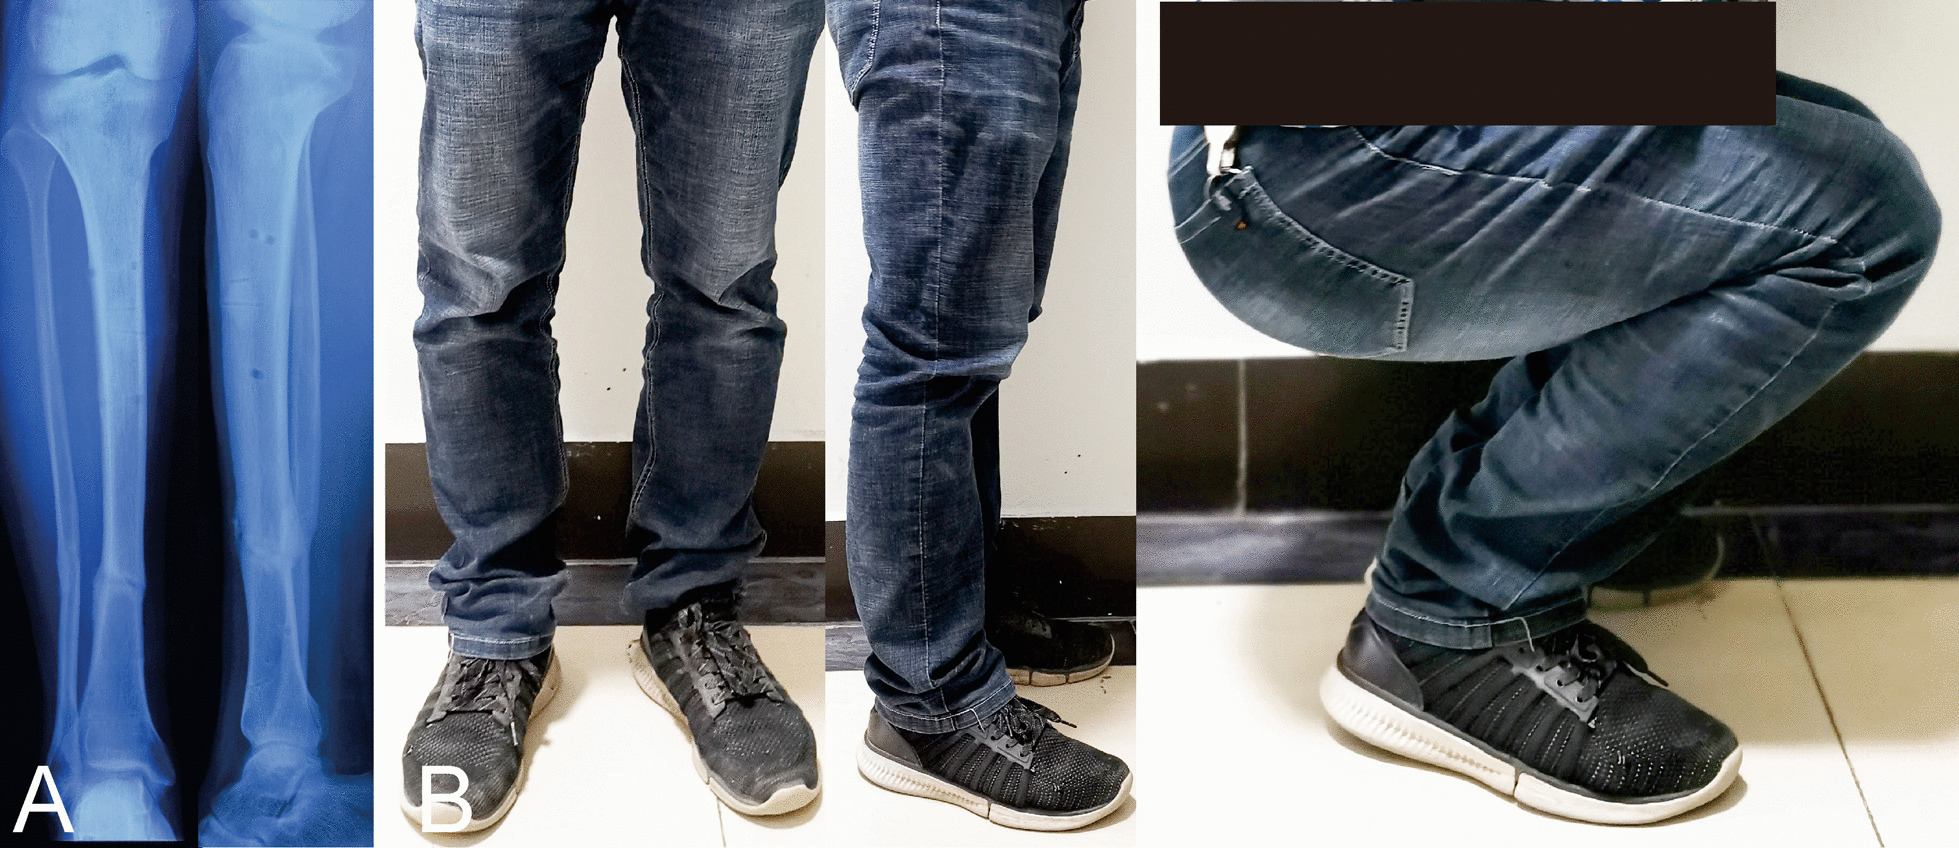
Follow-up images of the same patient after removing the HEF. A Radiographs six months later. B Clinical images of the patient, obtained at 12 months after HEF removal
